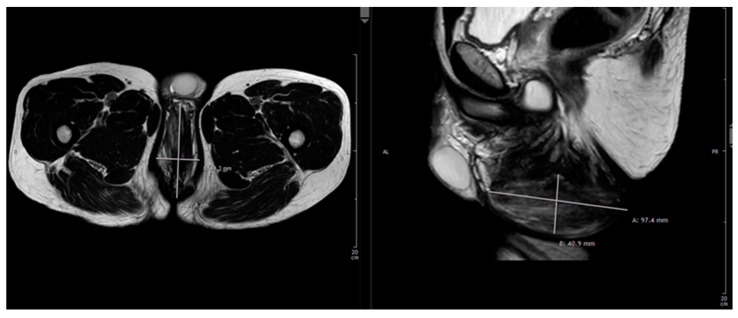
Diagnostic imaging: native and contrast enhanced T2-weighted MRI demonstrated a non-homogenous soft tissue proliferation with unclear demarcation to the surroundings, which was classified as a diffuse, partially fibrotic tissue transformation.
